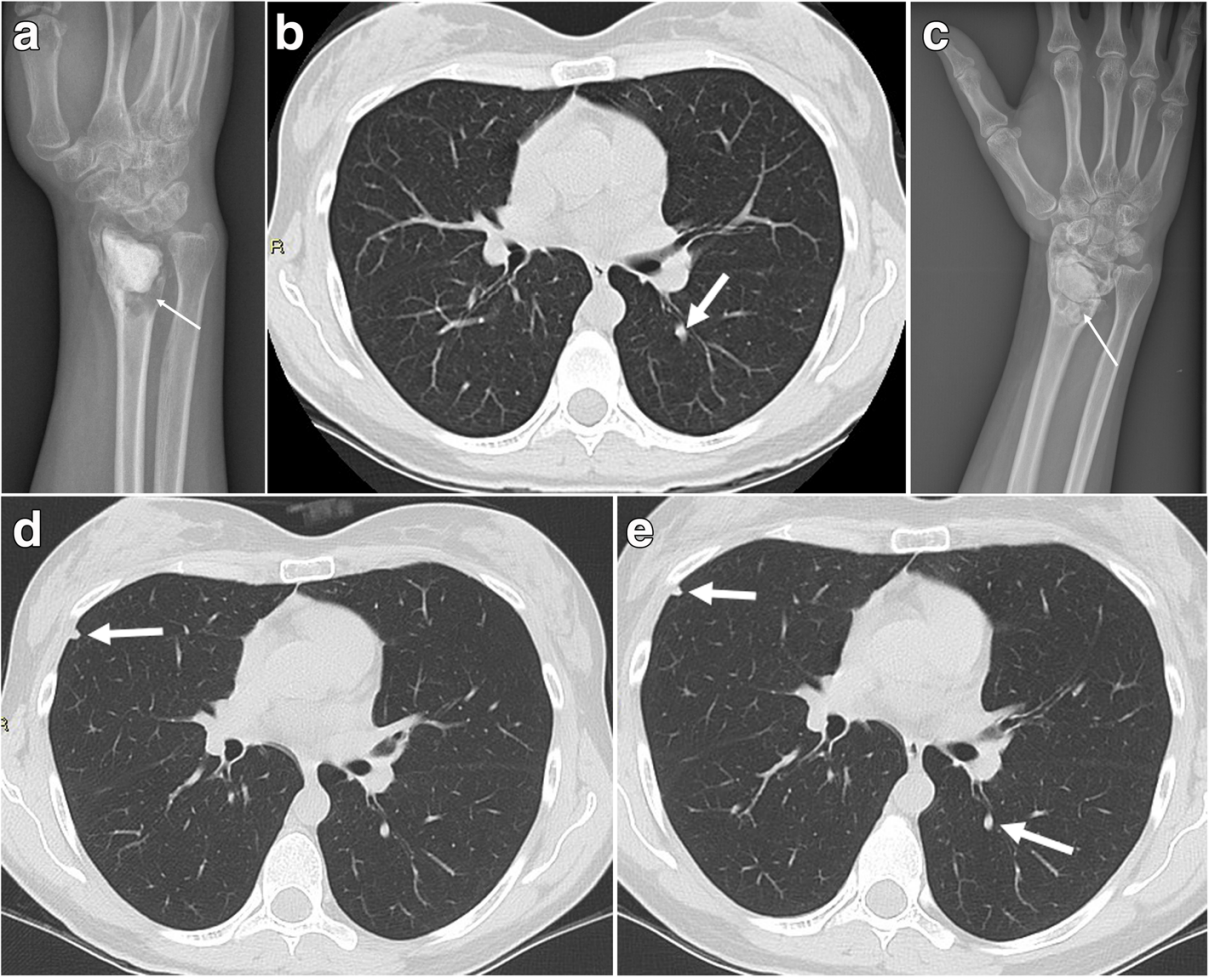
A case of giant cell tumor of bone and lung metastases treated with denosumab rechallenge. She was referred to our institute for local recurrence following curettage and cementing for distal radius giant cell tumor of the bone. Radiograph showed osteolytic lesions (arrow) surrounding the cement (a). Chest CT showed lung metastasis in the left lung (arrow) at presentation (b). She received preoperative denosumab therapy for 4 months and radiograph showed sclerotic formation (arrow) surrounding the cement (c). She then received curettage and cementing and postoperative denosumab therapy for 1 month. However, she experienced local recurrence 14 months after the operation and underwent an en bloc resection and reconstruction with an allograft. Follow-up chest CT showed a new lesion on the right lung (arrow) 3 years after the initial operation at our institute (d). She was treated with denosumab for 1 year and the lung lesions were stable (arrows) thereafter (e)
